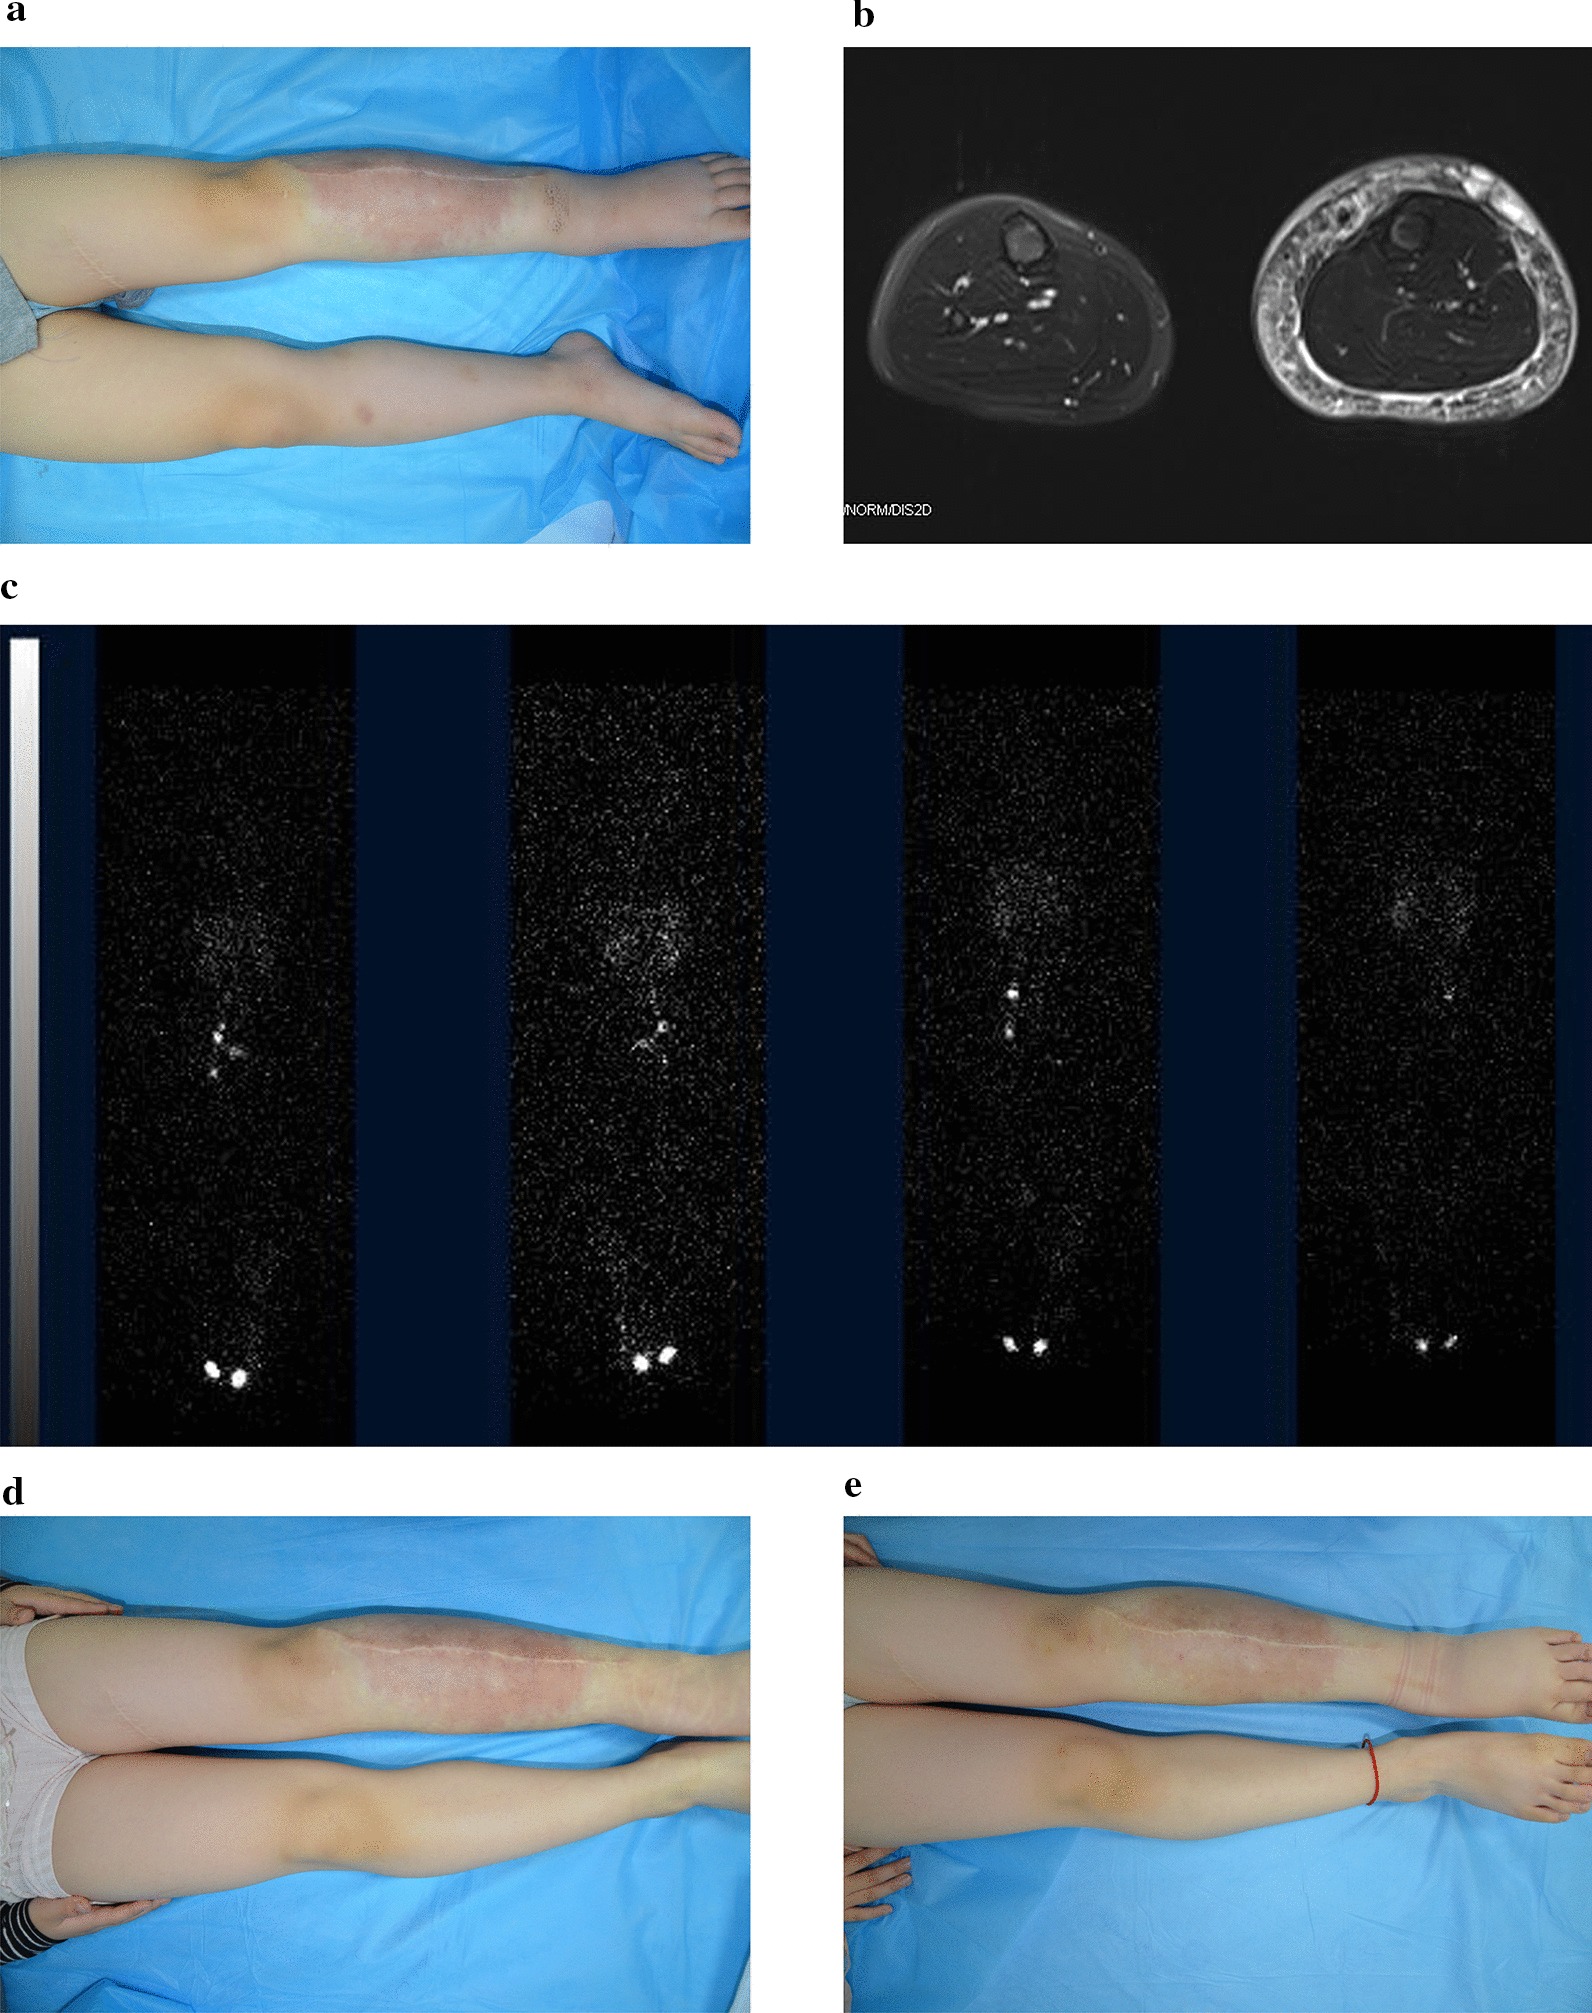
KHEs with chronic lymphedema involving the left lower extremity in a female. This patient’s vascular lesions were noted at 9 months of age. Limb swelling occurred 4 months later. She had partial resection of the thigh and leg KHEs at an outside institution at 2 years of age. a The photograph showed an ill-defined hyperpigmented plaque involving the left leg at referral (4 years old). Coronal (b) and horizontal (c) T2-weighted MRI revealed hyperintense lesions involving the dermis, subcutaneous tissue and deep fascia of the left leg. d Nuclear lymphoscintigram obtained 1 and 3 h after pedal injection showed uptake in the right pelvic and inguinal lymph nodes but not on the left side. Note the abnormal accumulation of tracer in the left thigh. e The patient received 12 months of sirolimus monotherapy. No alleviation of lymphedema was noted. Then, sirolimus was discontinued, and treatment with compression bandaging was initiated. g Twelve months after compression therapy
